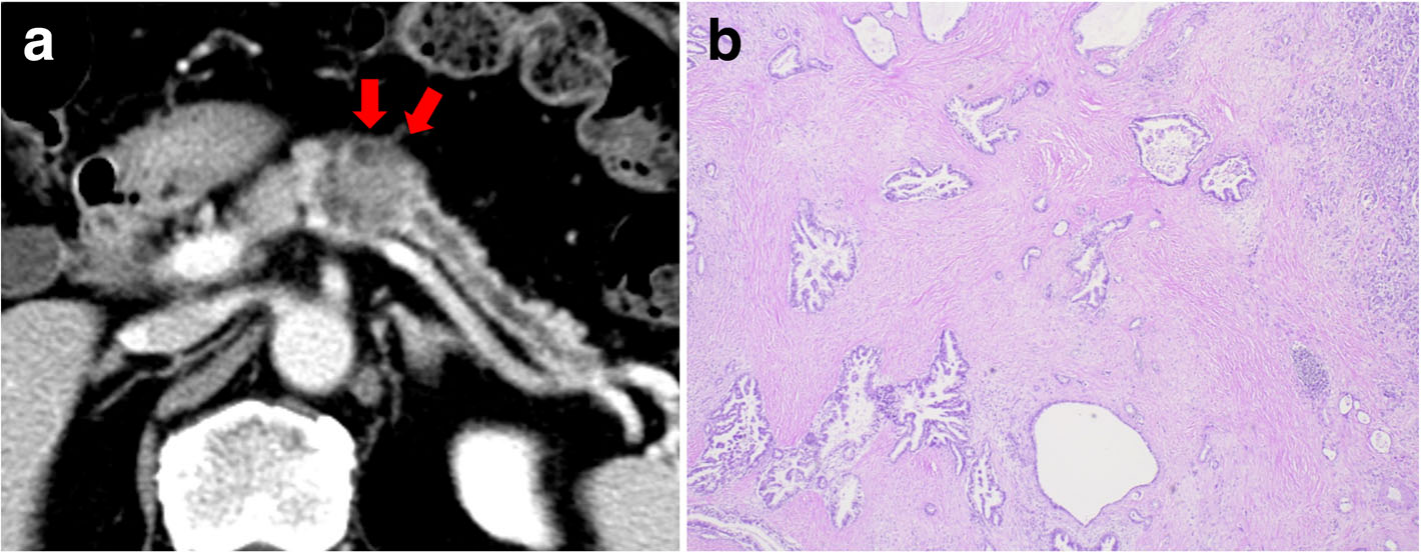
Primary operation for pancreatic body cancer. a Multi-detector row computed tomography (MDCT) showed pancreatic body cancer before the first surgery (arrows). b Pathological findings of the primary tumor were as follows: invasive ductal carcinoma, well-differentiated type, and (T3, N0, M0) stage IIa
